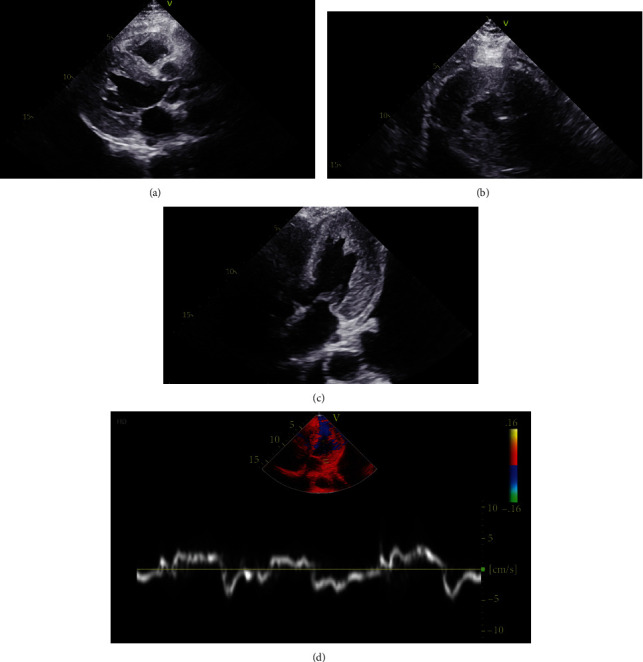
Echocardiogram (a). Parasternal long axis (b). Parasternal short axis (c). Apical two-chamber of left ventricle and (d) tissue Doppler showing severe left ventricular hypertrophy and diastolic dysfunction.
